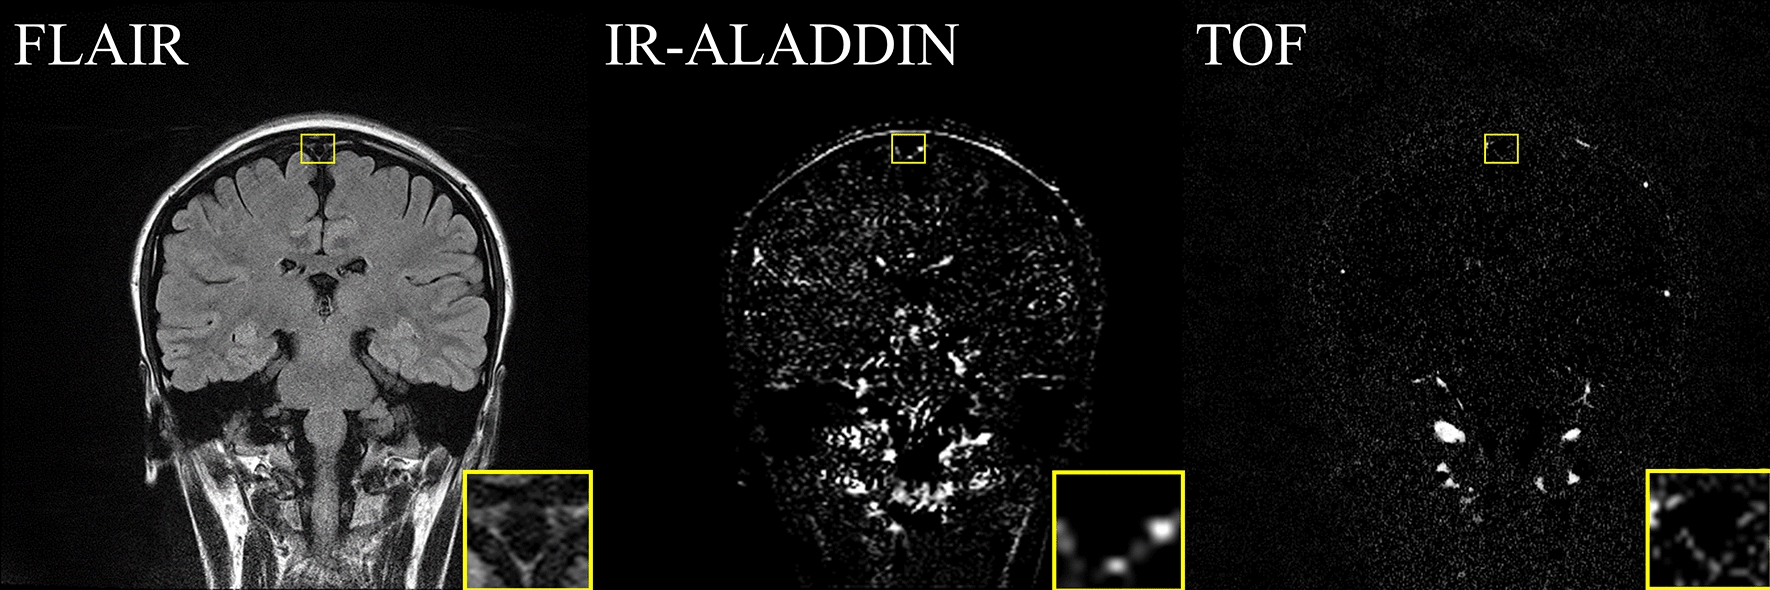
Comparison of mLVs images between FLAIR, IR-ALADDIN, and TOF in the same imaging position. Yellow boxes indicate the location of mLVs around the SSS
